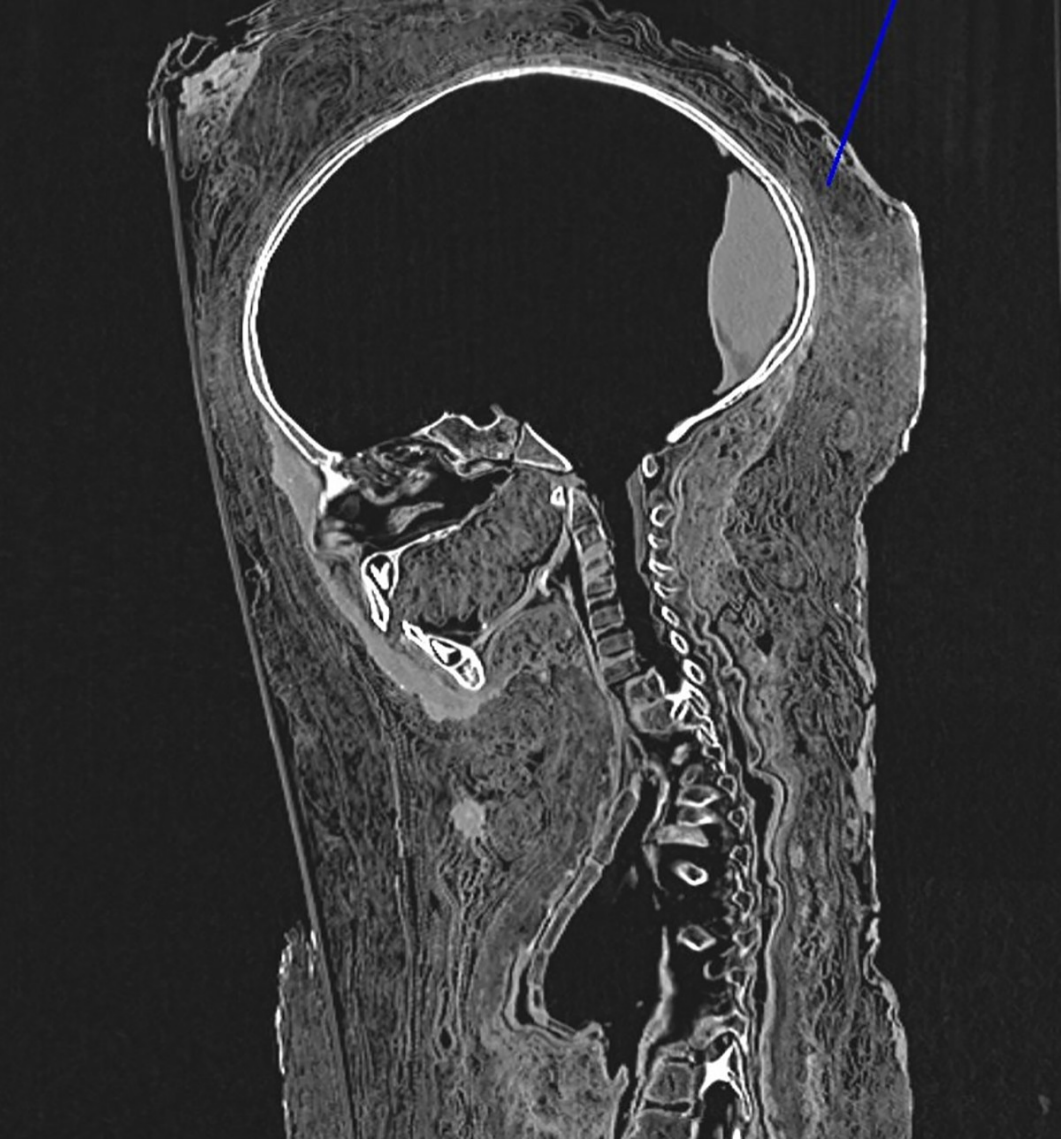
CT-scan section through skull and thorax. This shows the vertical plane of the embalming substance at the occipital skull cavity, the ethmoidal osseous defect and the linen filling of the mouth.
